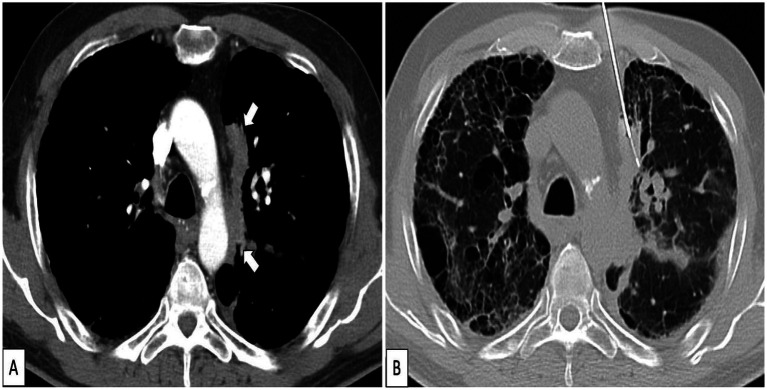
CT-guided percutaneous lung puncture. (A) Enhanced CT of the solid tissue adjacent to the left mediastinum (indicated by the arrow). (B) The CT-guided biopsy procedure was planned to minimize needle traversal of confluent emphysema by crossing the anterior mediastinum.
